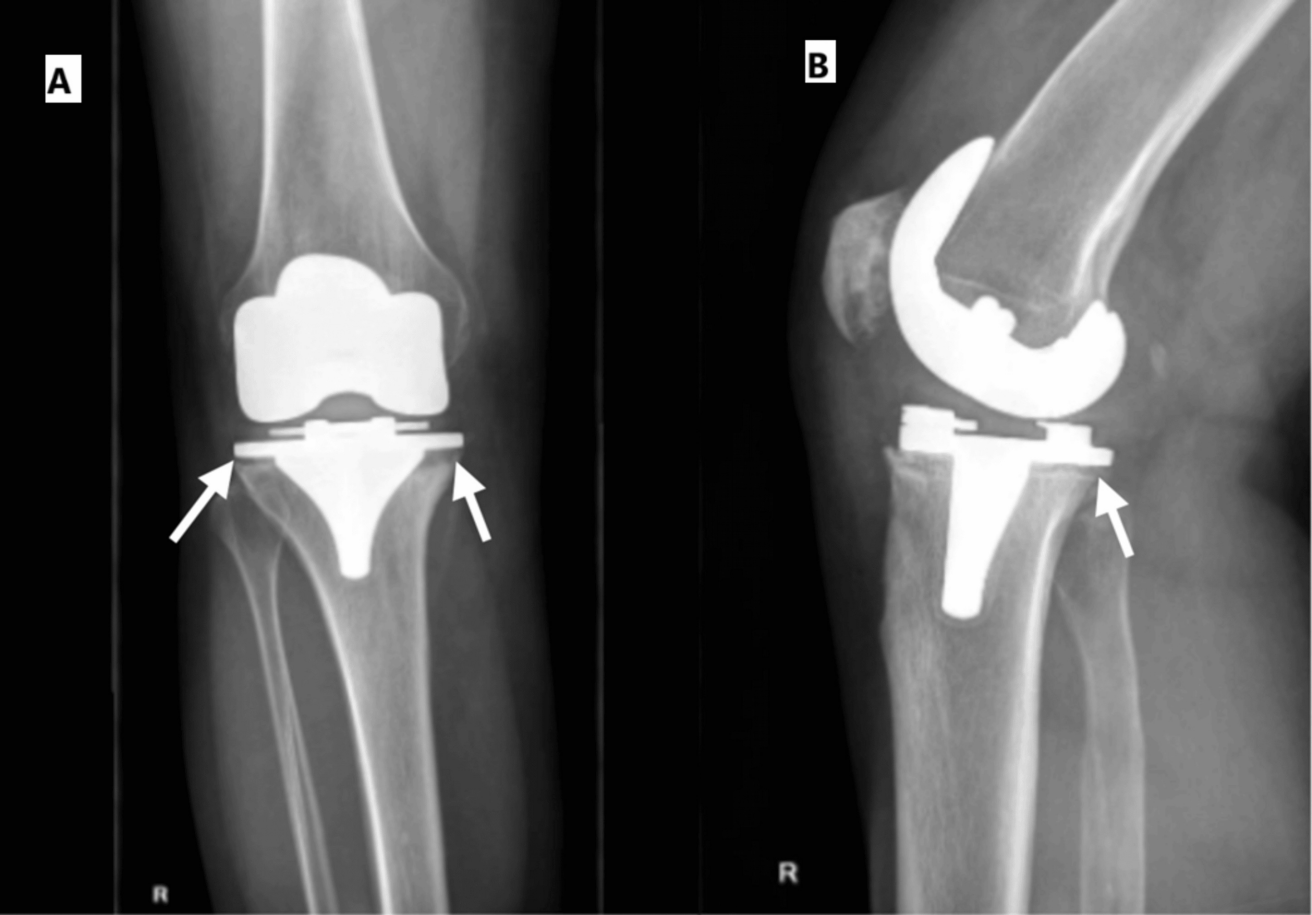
(A) Anteroposterior and (B) lateral radiographs of the right knee demonstrating loosening of the tibial component following total knee arthroplasty
